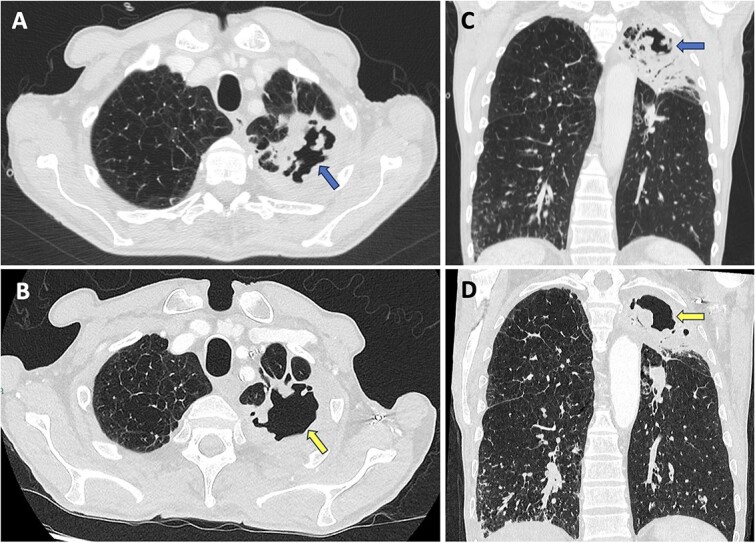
Images A and C are axial and coronal CT slices showing the cavity in the left upper lobe with surrounding consolidation (blue arrows). Images B and D are axial and coronal CT slices acquired 11 weeks later showing an increase in size of the cavity, with persistent surrounding consolidation (yellow arrows).
